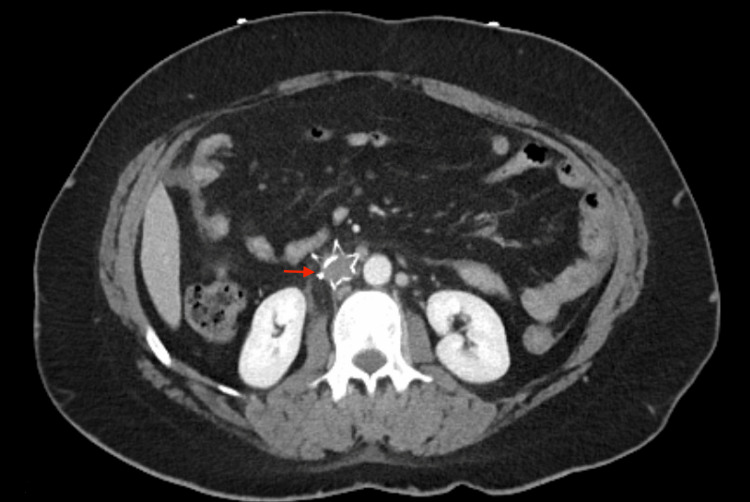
Axial CT angiography image of the lower extremity demonstrating the IVC filter. CT, computed tomography; IVC, inferior vena cava
